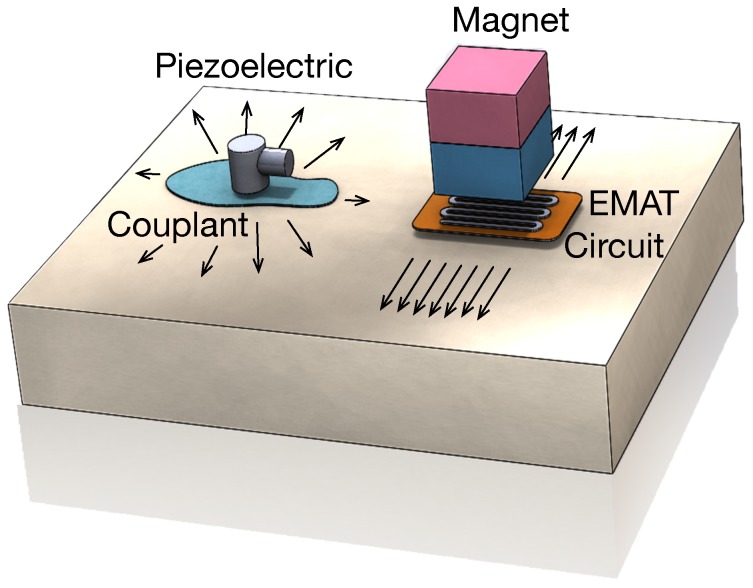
Conventional Ultrasound vs EMAT.
